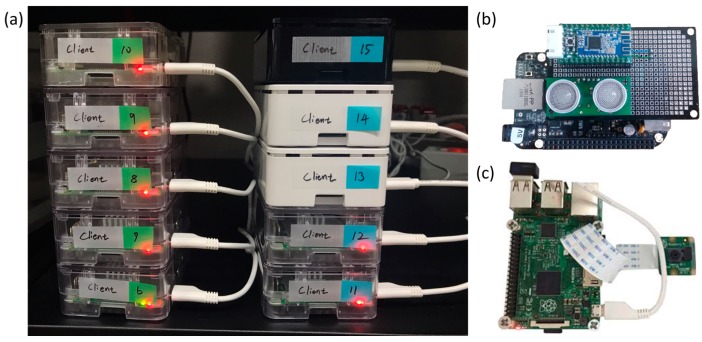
Experiment environment implemented using MinT. (a) client modules for experiment, (b) server module with ultrasonic sensor, (c) server module with camera sensor.
